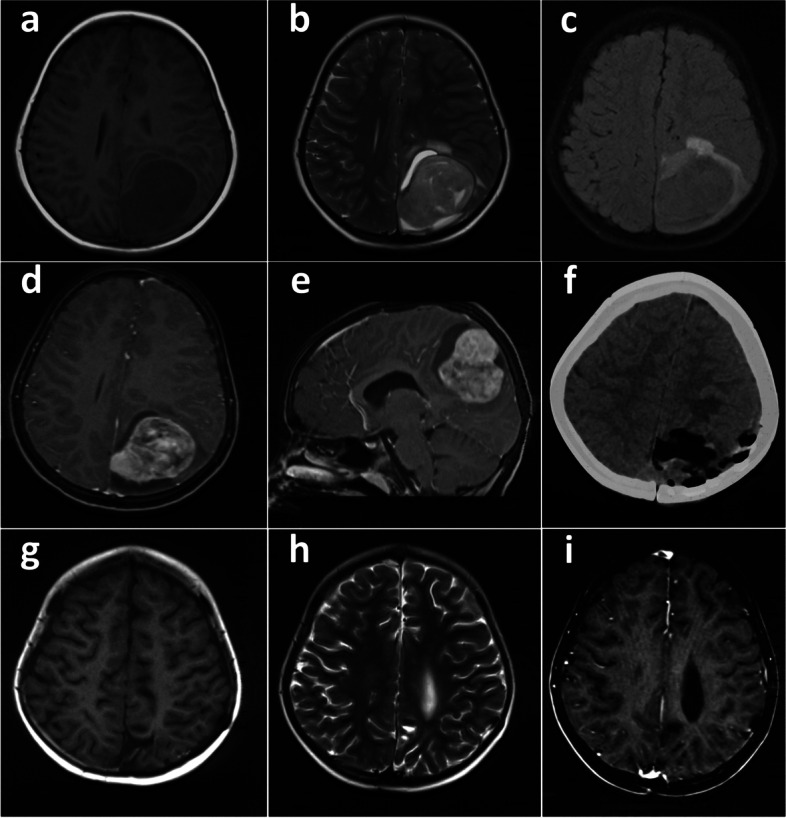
Preoperative and postoperative neuroimages of this DCS patient. Preoperative head MRI shows there is a heterogeneous intracranial mass. Axial T1-weighted MRI shows hypo-intense lesion in the left parietal lobe (a). Axial T2-weighted MRI shows inhomogeneous hyper-intense (b). Axial FLAIR image shows iso-intense (c). Axial and sagittal post-contrast T1-weighted MRI shows enhancement of the mass and the adjacent dura (d and e). Postoperative CT presents completely tumor resection (on the day after surgery) by axial CT (f). Postoperative MRI shows no tumor recurrence (19 months after surgery) which confirms on T1-weighted (g), T2-weighted (h), and axial coronal post-contrast T1-weighted (i)
